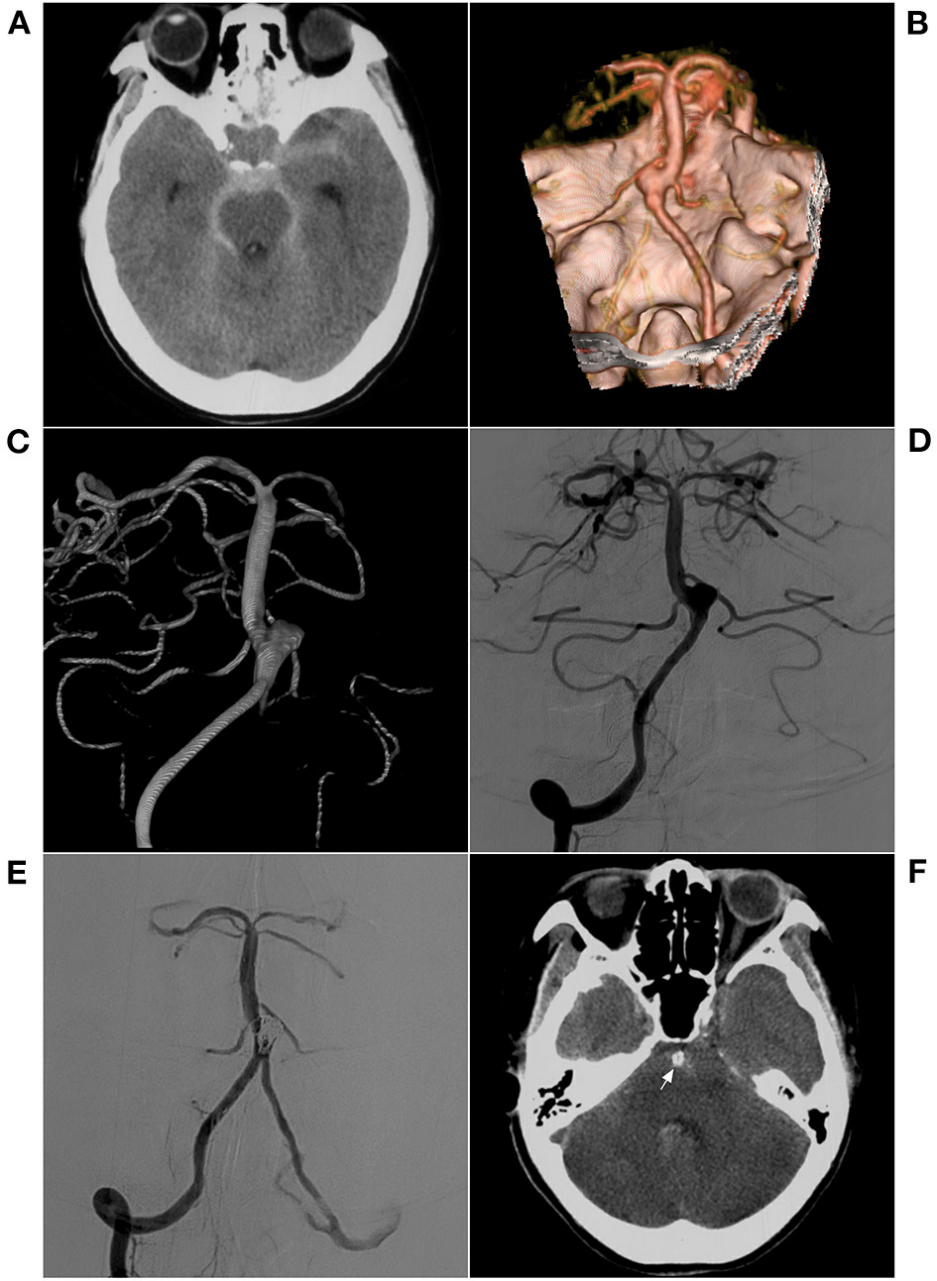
EVT with an ischemic complication. (A) CT shows hemorrhage in the anterior brainstem and subarachnoid space of the cisterna; (B) CTA shows a saccular wide-necked lateral-wall aneurysm in the inferior BA. (C,D) Preoperative VA-DSA (C for 3D reconstruction, D for 2D embolization) shows a saccular aneurysm in the inferior BA, lateral-wall type. (E) Postoperative VA-DSA shows the aneurysm following stent-assisted EVT, MRRC Grade I. (F) For the patient who immediately developed coma after EVT, the postoperative CT shows no hemorrhage and the coil (arrow) in the BA lumen. BA, basilar artery; CT, computed tomography; CTA, CT angiography; DSA, digital subtraction angiography; EVT, endovascular therapy; MRRC, Modified Raymond-Roy Classification; VA, vertebral artery.
